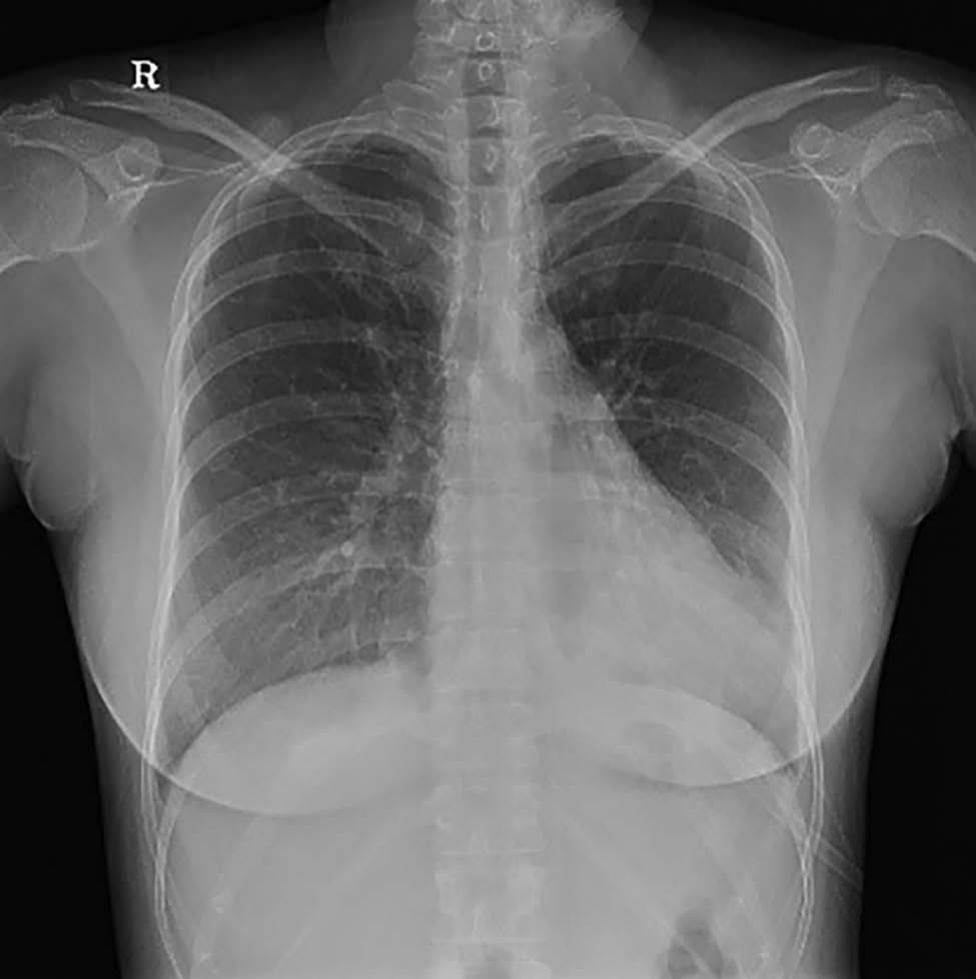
Chest X-ray POD 4 decreased BLL haziness, but remained. POD = postoperative day.
